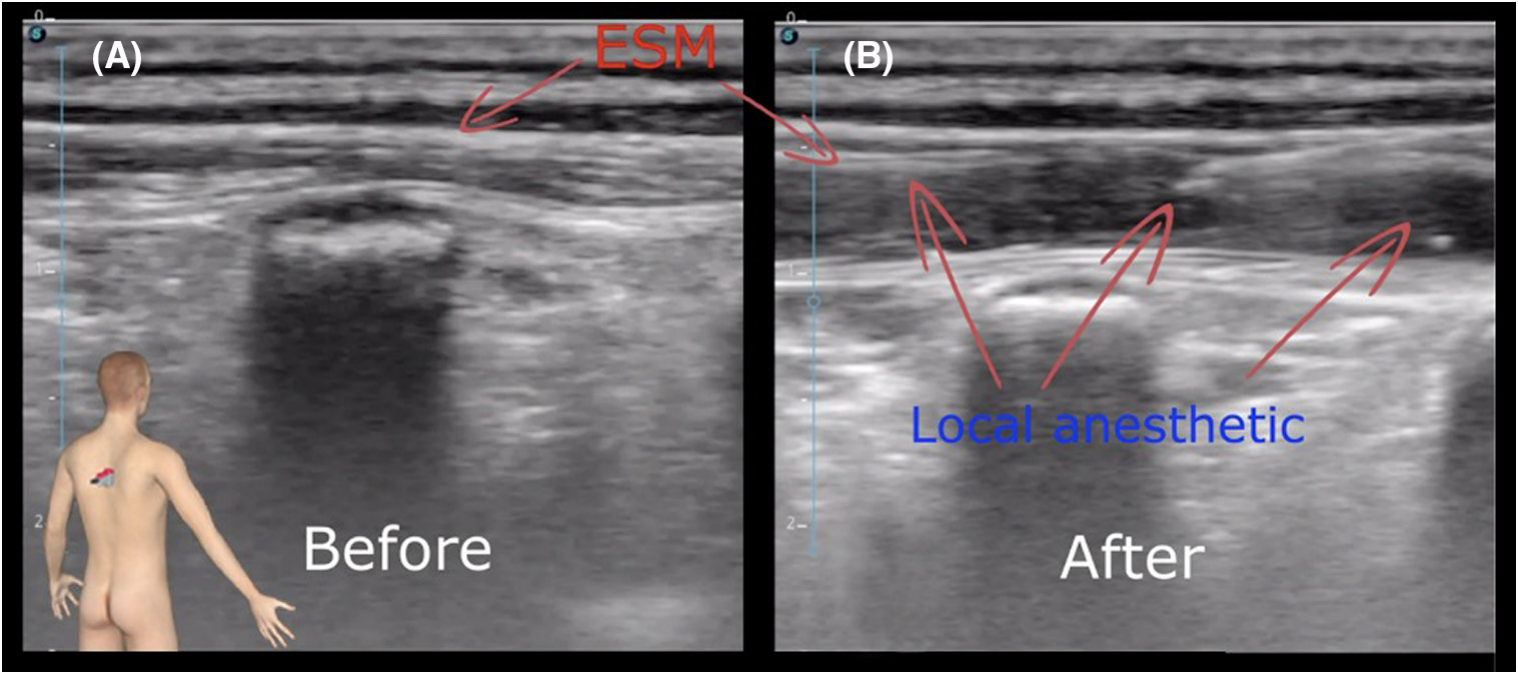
Ultrasound‐guided Erector Spinae Plane Block using a high‐frequency linear transducer (3–16 MHz) ((A): before injection, (B): after injection, ESM: Erector spinae muscles).
